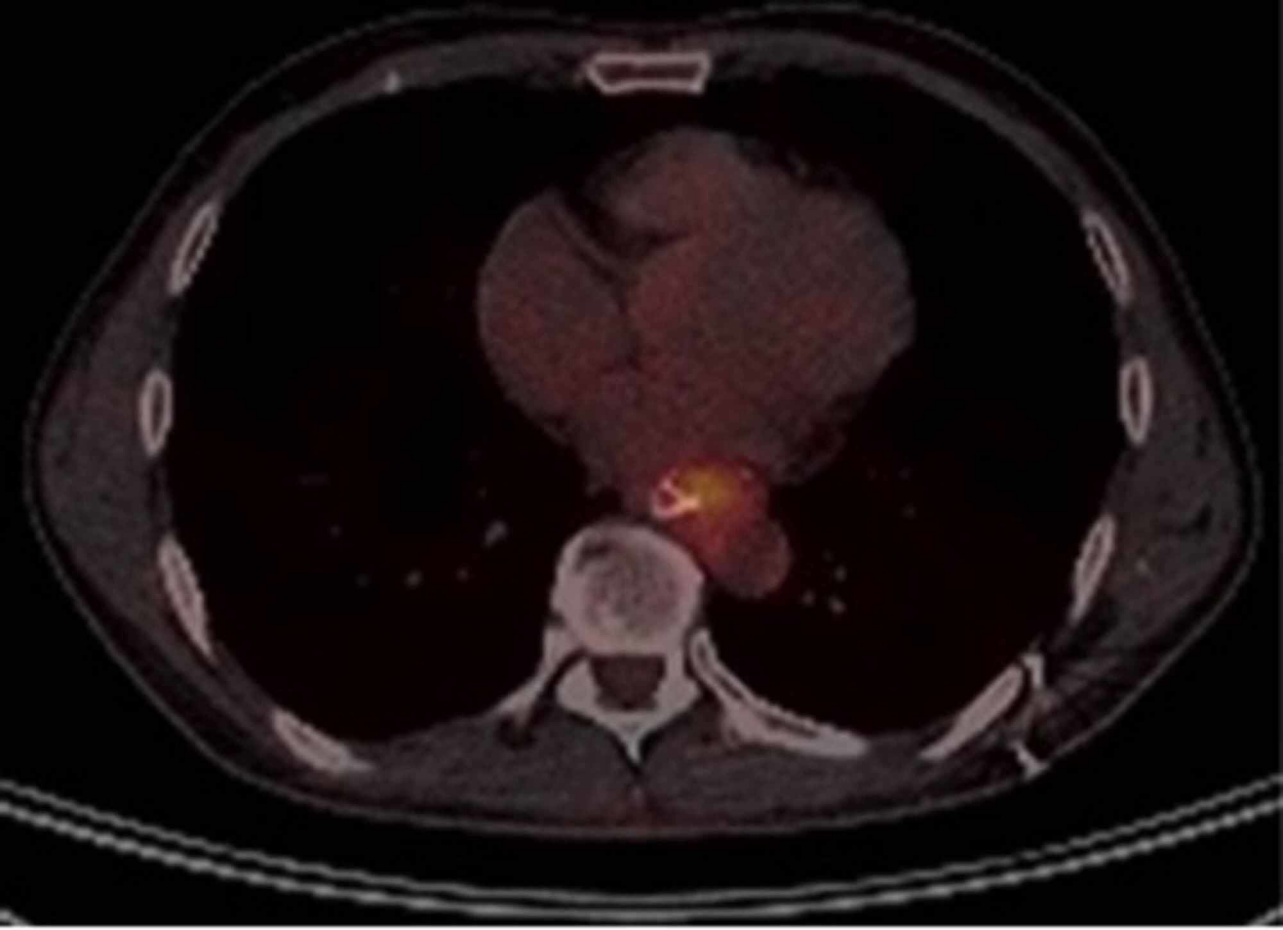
PET-CT with hypermetabolism in the esophagus-jejunal anastomosis due to relapse of a known primary tumor
